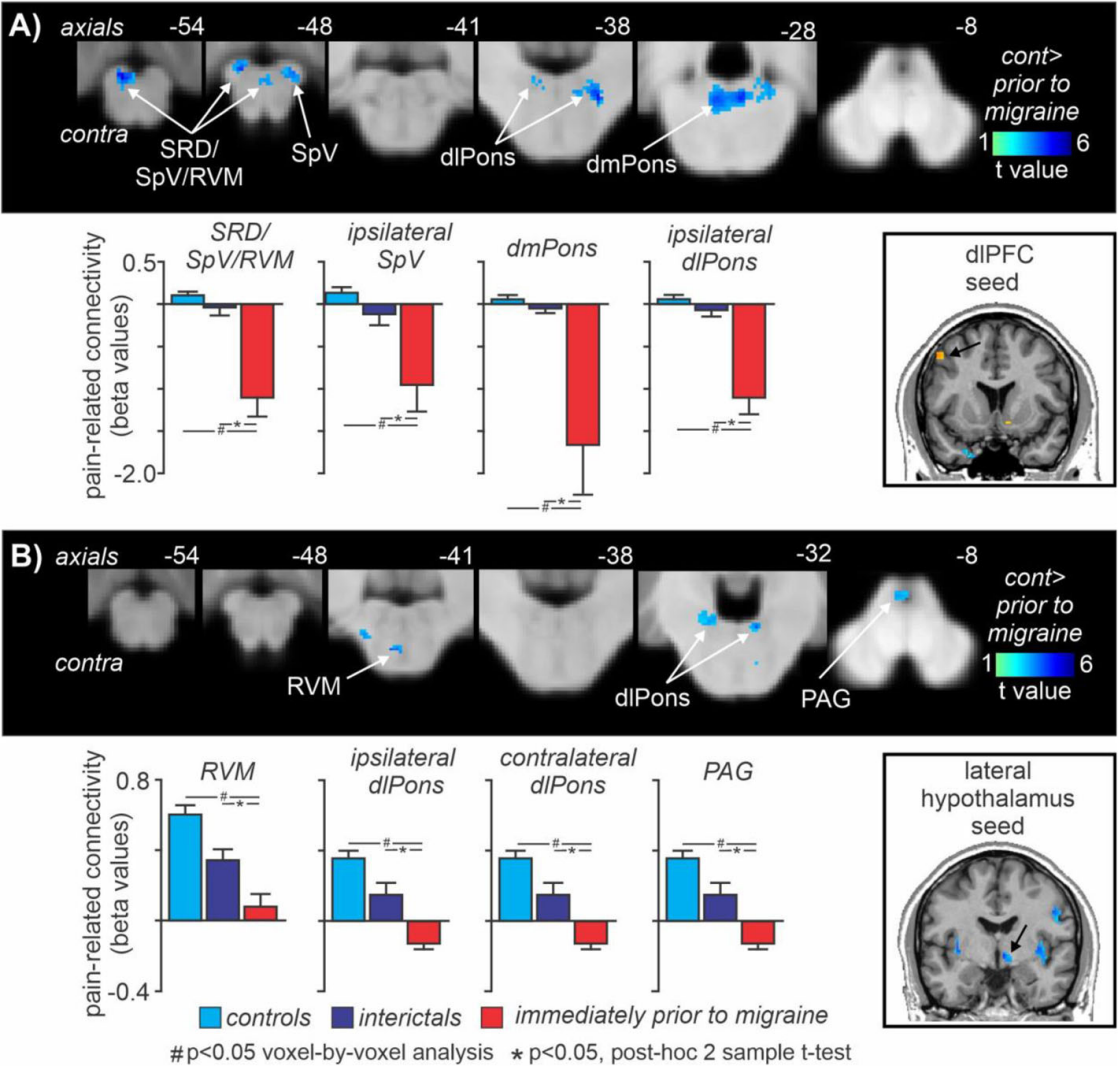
Pain-related connectivity: Significant differences in (A) contralateral dorsolateral prefrontal cortex (dlPFC) and (B) ipsilateral hypothalamus acute pain-evoked changes in connectivity (psychophysiological interaction analysis) between controls and migraineurs in the period immediately prior to a migraine headache. Significant clusters are overlaid onto a mean T1-weighted brainstem template image. Slice locations in Montreal Neurological Institute space are indicated to the top right of each slice. The dlPFC and hypothalamic seeds are shown in the lower right inset. Note that dlPFC pain-related connectivity strengths were significantly reduced in a number of brainstem regions including the dorsomedial pons (dmPons), dorsolateral pons (dlPons), spinal trigeminal nucleus (SpV), and a cluster encompassing the nucleus reticularis dorsalis (SRD)/SpV and rostral ventromedial medulla (RVM). More restricted pain-related hypothalamic connectivity changes occurred in the dlPons, RVM and also in the region of the midbrain periaqueductal gray matter (PAG). Plots of mean (±SEM) beta values (effect sizes) revealed that pain-related changes in connectivity decreased significantly in migraineurs only during the 24-h period immediately prior to migraine, that is they were not different between controls and migraineurs during the interictal phase
